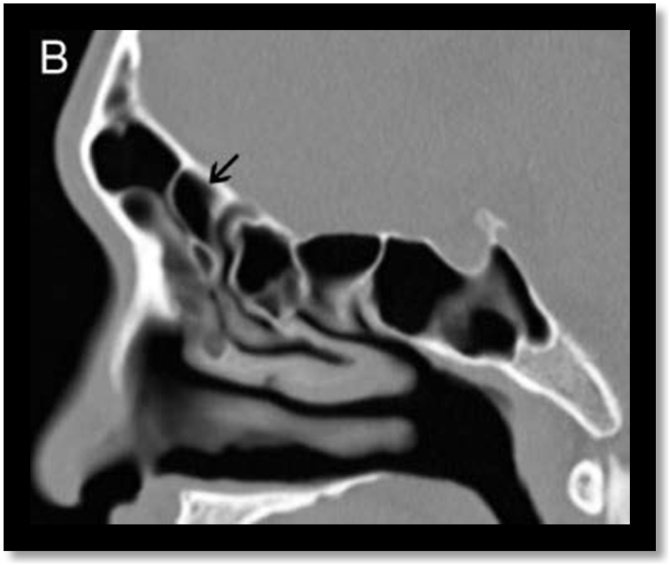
Supra bulla frontal cell. Sagittal computed tomography section demonstrates a supra bulla frontal cell (black arrow).
